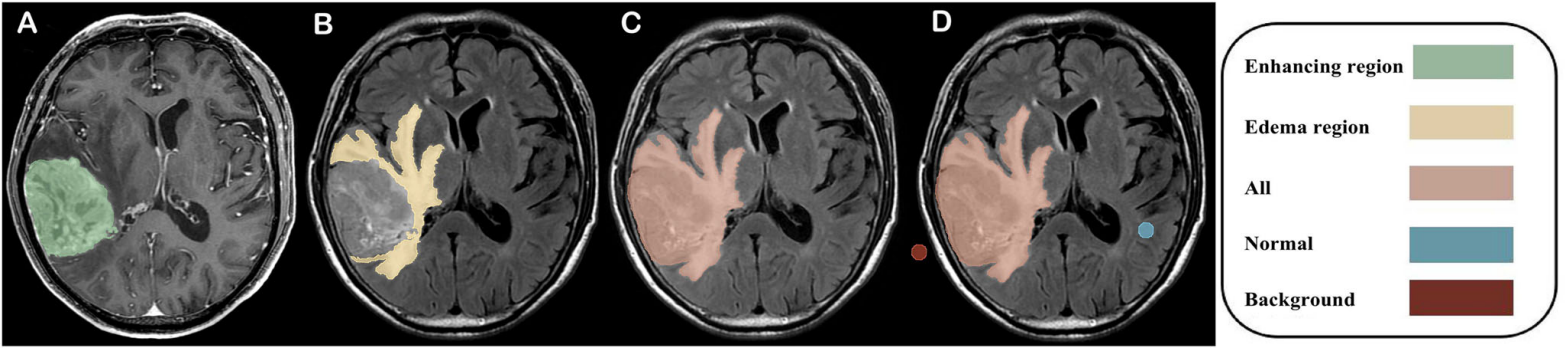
Representative case of *pTERTm* GBM. A 74‐year‐old male patient presented with a glioblastoma in the left parietal lobe. (A) The standard deviation of FLAIR signal intensity (FLAIR_SD_) in the enhancement region was 152.37. (B) In the edema region, FLAIR_SD_ and relative FLAIR (rFLAIR) were 148.30 and 2.06, respectively. (C, D) The FLAIR signal intensities of the whole lesion, contralateral normal region, and background were 355.81, 193.73, and 3.49, respectively. Nomogram analysis yielded a total score of 80, corresponding to a predicted probability of *pTERT* mutation of 0.97.
